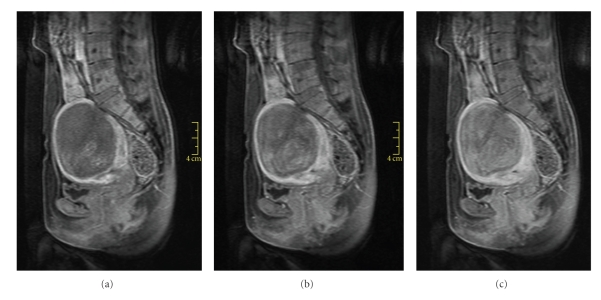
Contrast-enhanced sagittal T1-weighted screening MR dynamic images acquired at 30, 60, and 90 seconds post contrast injection (from left to right). Fibroid shows lower enhancement than the myometrium in the first 2 minutes postinjection.
